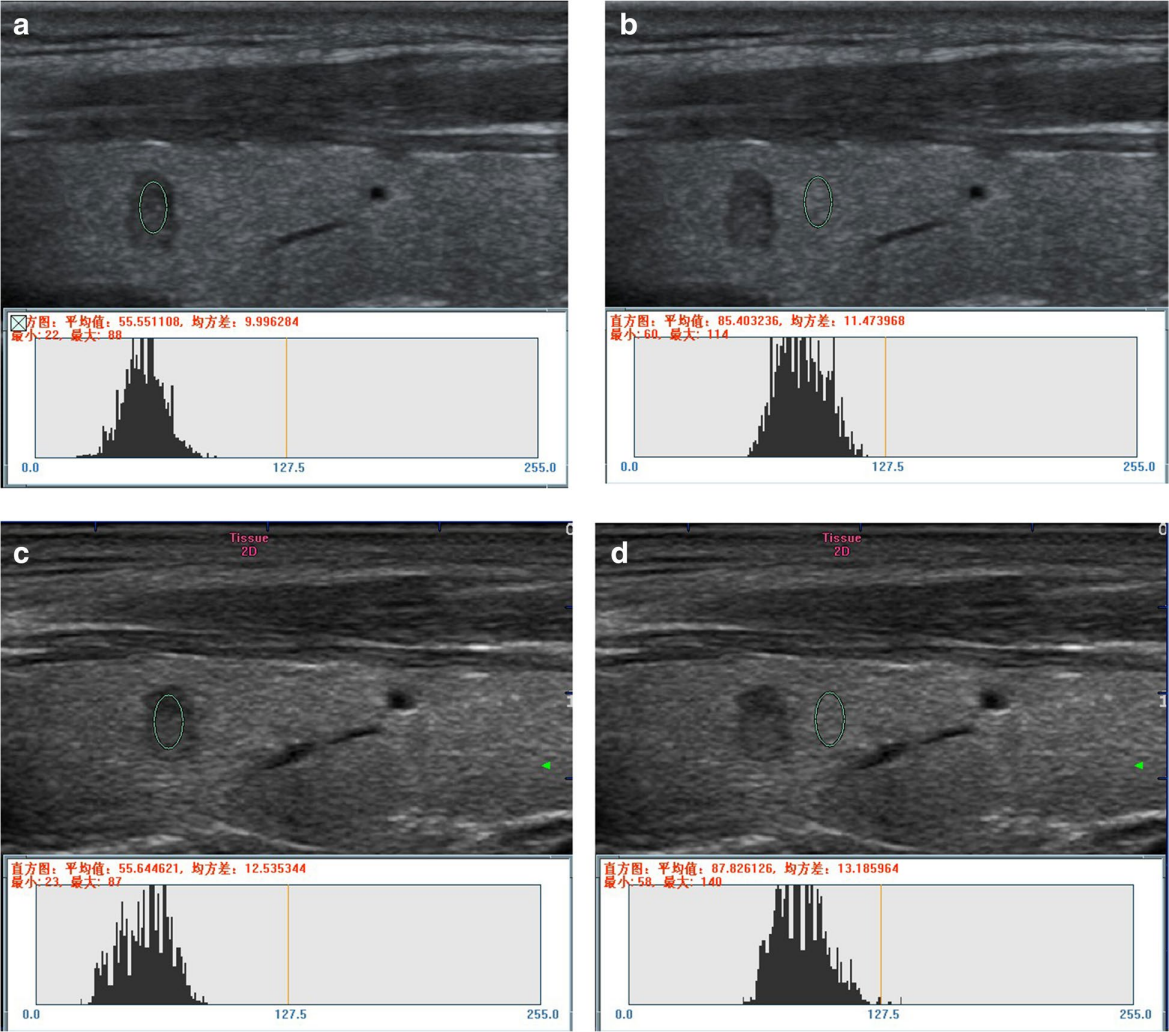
Measurement of the UGSR value for PTMC on the right lobe of the thyroid. a and b (ultrasonic apparatus: Mindray Resona7): In the outpatient examination group, a longitudinal scan revealed grayscales of 55.551108 and 85.403236 for PTMC and thyroid tissues at the same gain level, respectively. The UGSR value was 0.650457 (55.551108/85.403236). c and d (ultrasonic apparatus: MyLab Classic C): In the preoperative positioning group, a longitudinal scan showed grayscales of 55.644621 and 87.826126 for PTMC and thyroid tissues at the same gain level, respectively. The UGSR value was 0.633577 (55.644621/87.826126), and the interval was seven days. The difference of the UGSR was 0.016880 (0.650457–0.633577) between the two groups (the outpatient examination and the preoperative positioning groups)
